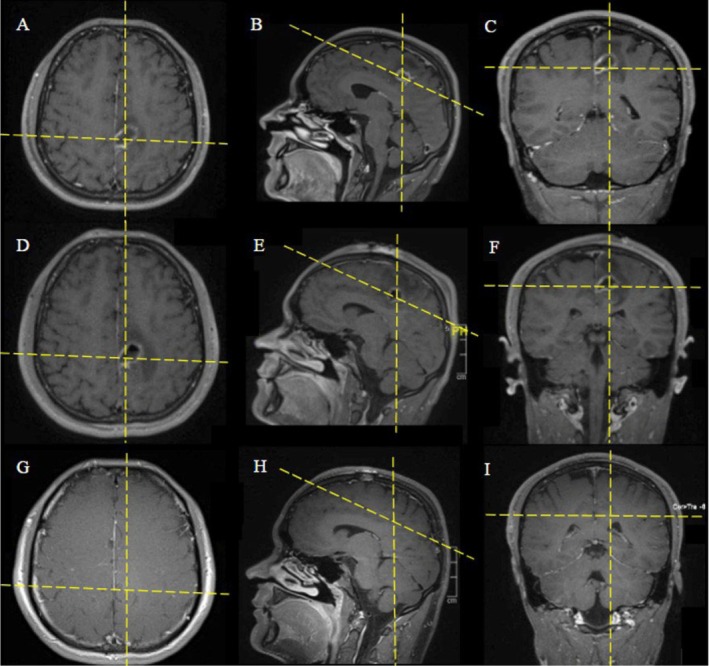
MRI evolution of left perirolandic paramedian brain abscess (T1‐weighted fat‐saturated post‐contrast): Preoperative axial/sagittal/coronal (A–C) show a 2.4 × 1.4 cm thin rim–enhancing lesion with surrounding vasogenic edema. Postoperative day 1 (D–F) demonstrates persistent rim enhancement with slightly increased edema. Four‐month follow‐up (G–I) shows near‐complete resolution with only minimal residual enhancement.
